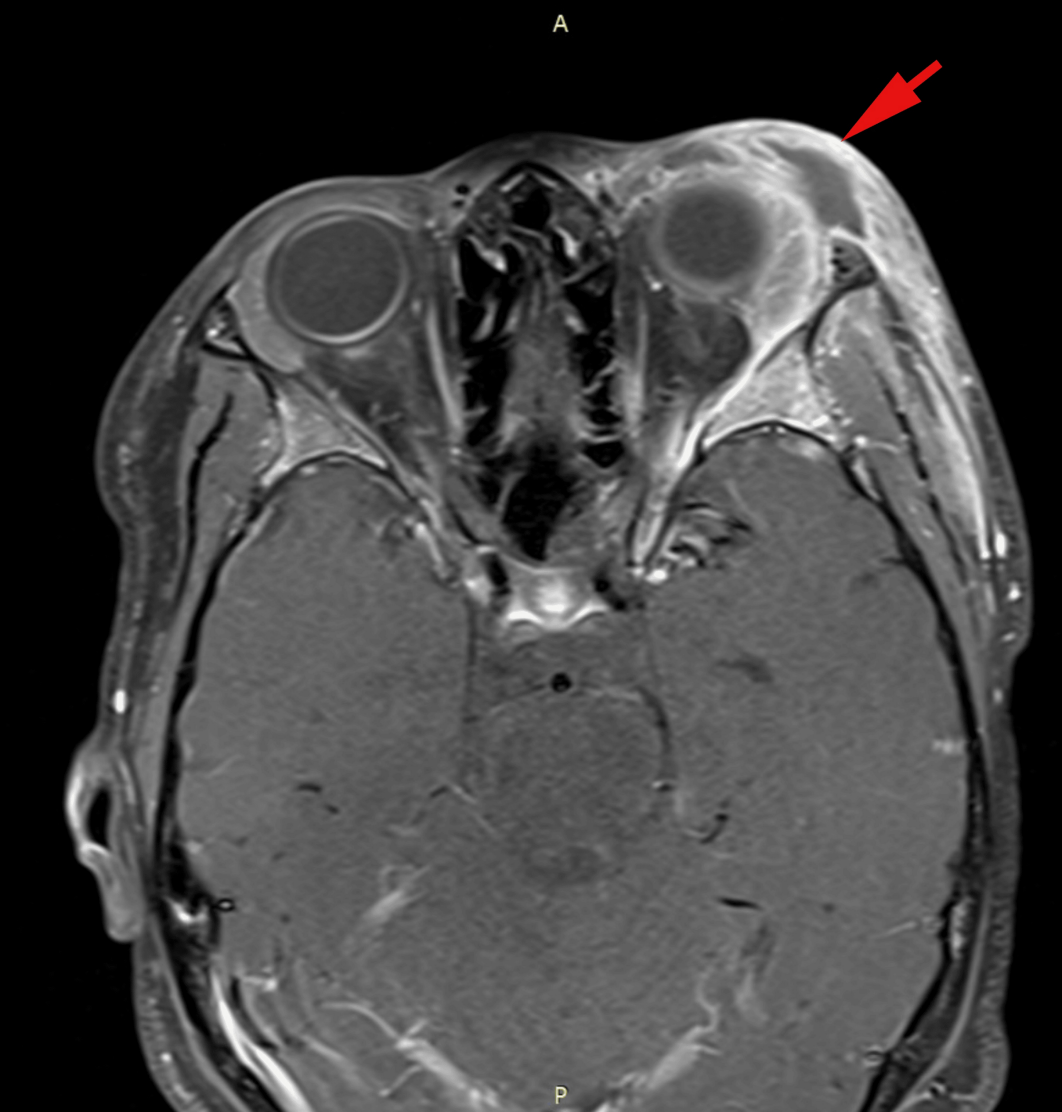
Post-contrast axial T1 fat-suppressed MRI of the orbits Axial T1 fat-suppressed post-contrast MRI showing a left frontal epidural abscess with anterior midline involvement and increased signal intensity. The abscess measures approximately 1.7 cm x 0.9 cm in the transverse plane and 3.8 cm in the cephalocaudal plane, causing posterior displacement of the superior sagittal sinus. Dural thickening and enhancement in the anterior frontal region are noted, consistent with pachymeningitis. Left orbital abscesses in the superior eyelid and postseptal extraconal space are visible, along with periorbital soft tissue swelling and slight left-sided proptosis.
